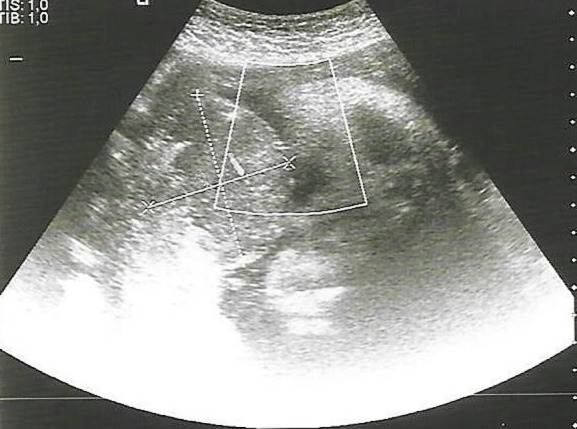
sonogram obtained at 34 weeks and 6 days´ gestation shows the chorioangioma measuring 6.27 x 5.38cm
